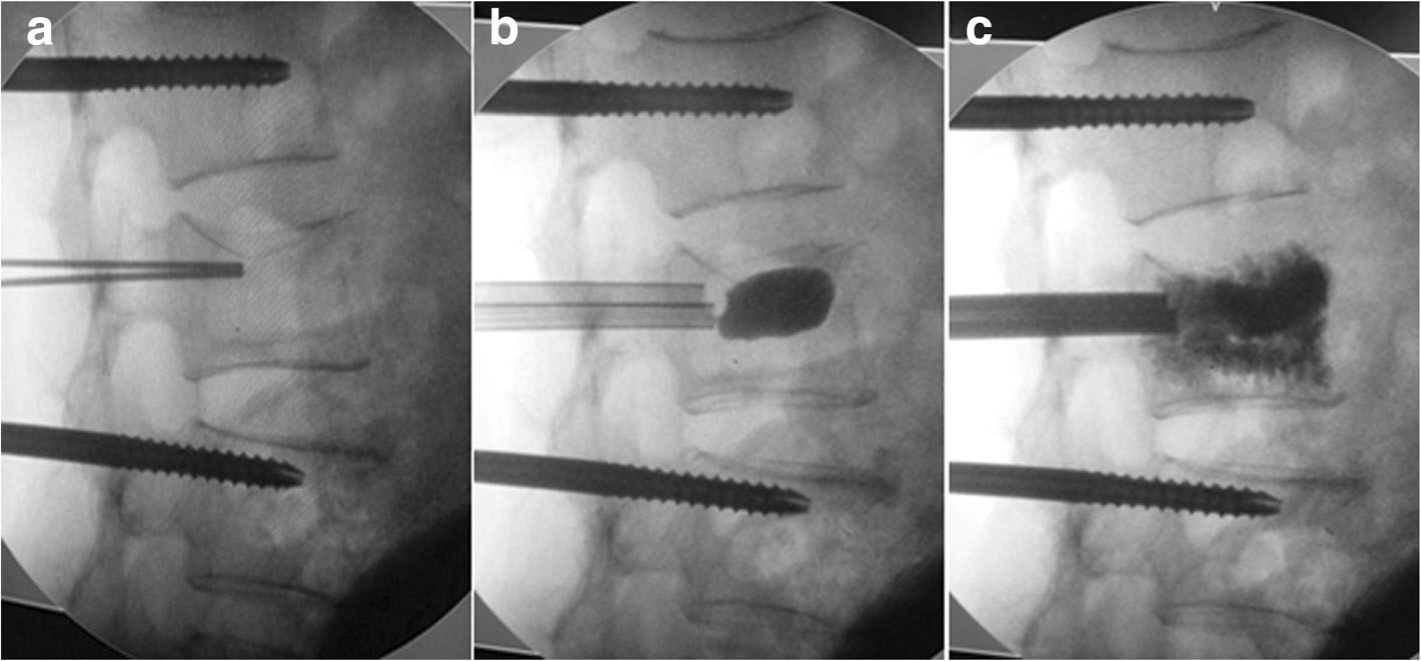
Intraoperative radiographs. a Distracting reduction using PIVR. b The balloon slowly inflates by injecting contrast media. c Bone cement being injected into the collapsed vertebral body
